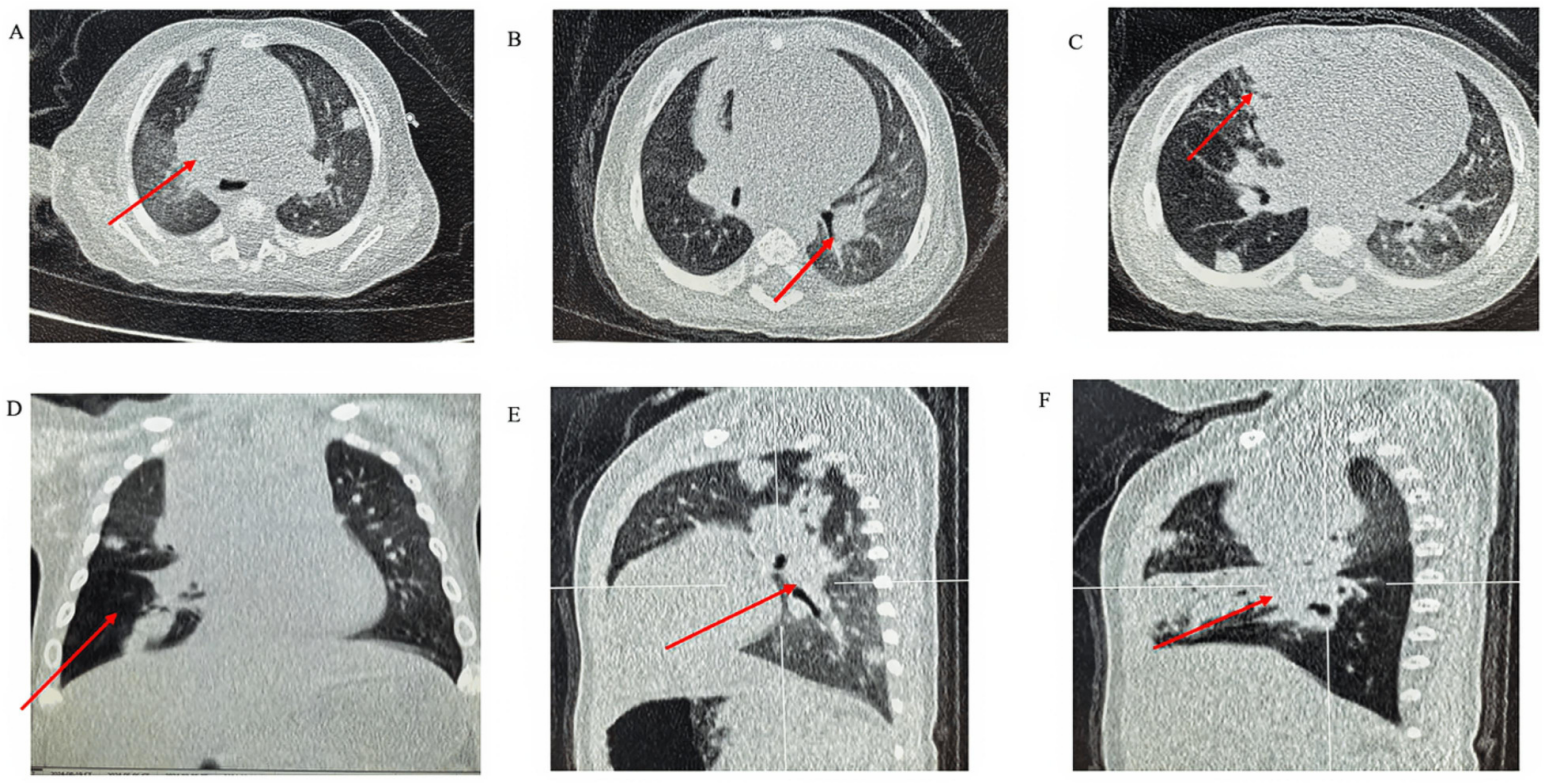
(A–C) Axial CT views demonstrating airway obstruction. (D–F) Coronal and sagittal reconstructions showing emphysematous changes and persistent nodular lesions.
